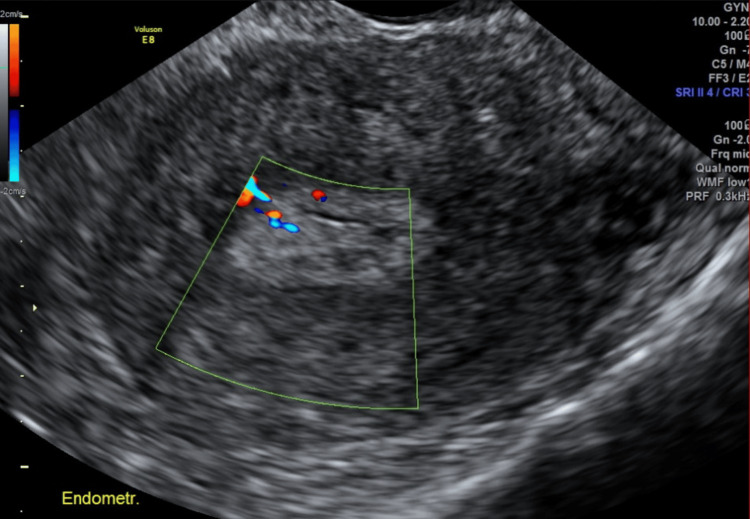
The hyperechoic oval mass observed on ultrasound with a feeding vessel is characteristic of high vascularity seen in some sarcomas.
